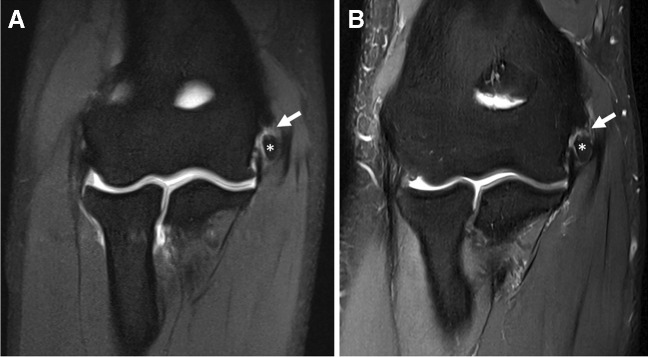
Thrower’s elbow. Twenty-two-year-old man with medial elbow pain and suspected ulnar collateral ligament tear. A Coronal T1-weighted fat-suppressed MR arthrogram image at the time of injury shows an ulnar collateral ligament tear at the humeral attachment (arrow) with adjacent ossicle (asterisk). B Coronal intermediate-weighted fat-suppressed conventional 3T MR image three months later shows the tear (arrow) and ossicle (asterisk) to similar advantage
